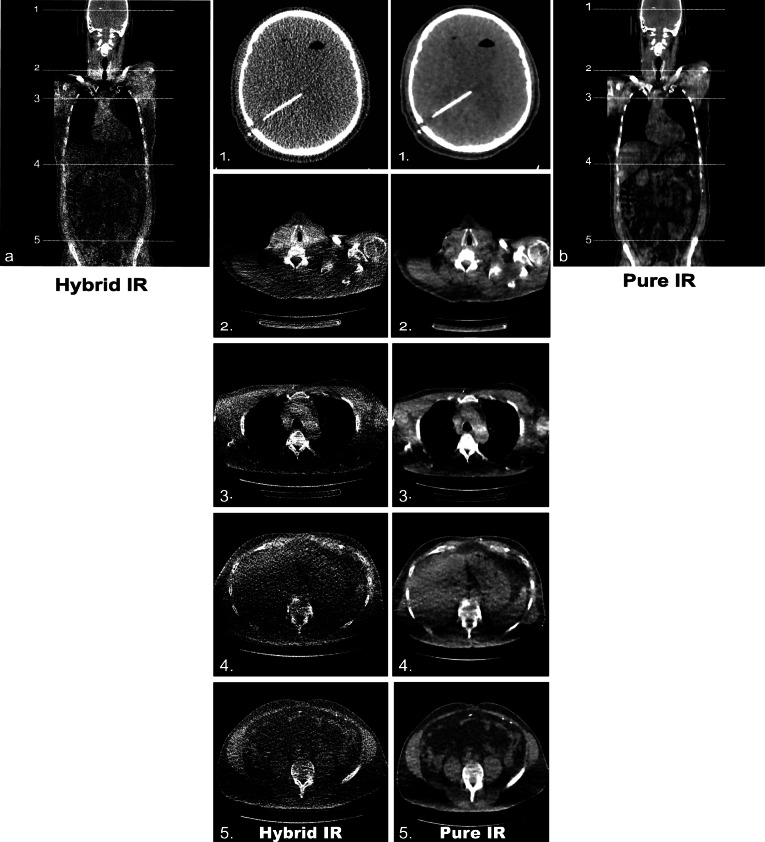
Image quality comparison for ULD CT: hybrid *versus* pure IR. ULD CT coronal (3-mm thickness) images reconstructed with hybrid IR (a) and pure IR (b). Representative axial (3-mm thickness) images at five anatomical levels (1, head; 2, neck; 3, thorax; 4, abdomen; 5, pelvis) are shown for hybrid IR (left) and MBIR (right). Images were visualised using soft-tissue window settings (window width 400 HU; window level 40 HU). *ASIR* Adaptive statistical iterative reconstruction, *CT* Computed tomography, *FBP* Filtered back projection, *IR* Iterative reconstruction, *MBIR* Model-based iterative reconstruction, *ULD* Ultralow dose
